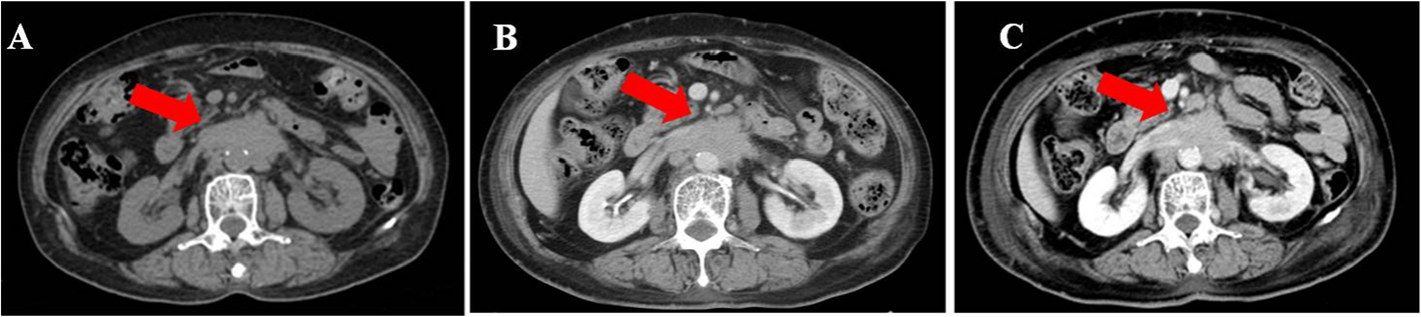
Abdominal CT showing the metastatic para-aortic lymph node. Notes: a pretreatment of Olaparib. b Six months posttreatment of Olaparib. c Nine months posttreatment of Olaparib
